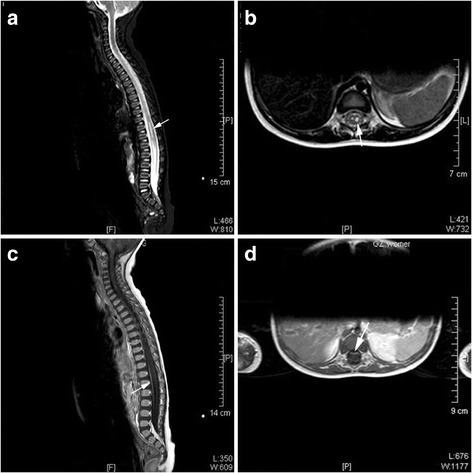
Abnormal MRI signal in the thoracic spinal cord. The spinal cord at the T10-T12 levels appeared a little swollen, with strip-liked abnormal signal in the bilateral anterior horn regions on the sagittal (a, c) and axial (b) views. This change was more obvious on the left side. The lesions are hyperintense on T2-WI and hypointense on T1-WI. There was no change on T1-enhancement of the lesions. Contrast-enhancement on the axial T1-WI showed strong enhancement of the ventral root (d)
